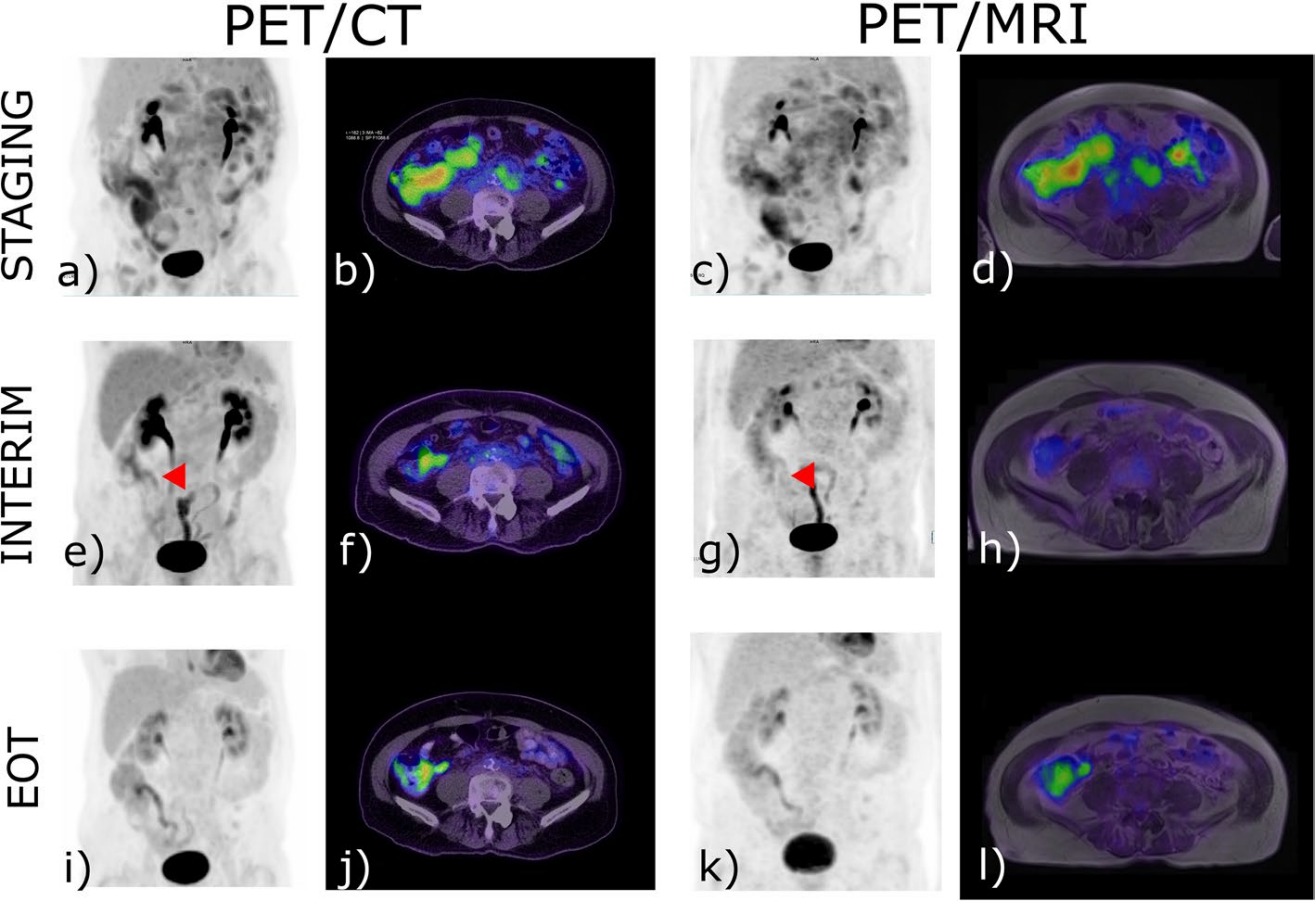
A 68-year-old male with biopsy proven Mantle cell lymphoma of the terminal ileum, stage IVA. At Baseline PET/ CT (a,b) and PET/MR (c,d) there was concordant avidity above that of background hepatic activity within the terminal ileum. At interim re-staging there remained uptake within the terminal ileum identified on the PET/CT (e,f) but not on the PET/MR (g,h) – red arrowhead. At end of treatment (EOT), there remained concordant persistent uptake in the terminal ileum. SUV scale set between 0—8
